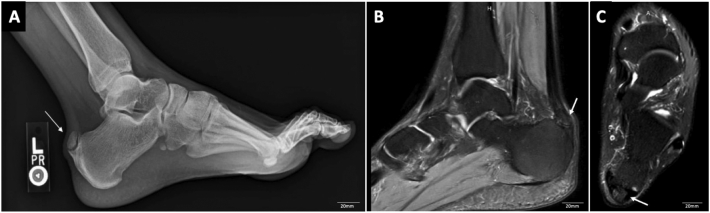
(A) Weightbearing lateral radiograph of the left ankle demonstrating presence of a large calcific ossicle at the posterosuperior aspect of the calcaneus (white arrow). Sagittal (2B) and axial (2C) T2-weighted magnetic resonance imaging of the left ankle revealing an ossicle at the posterosuperior aspect of the calcaneus in the tendinous insertion of the Achilles' tendon.
